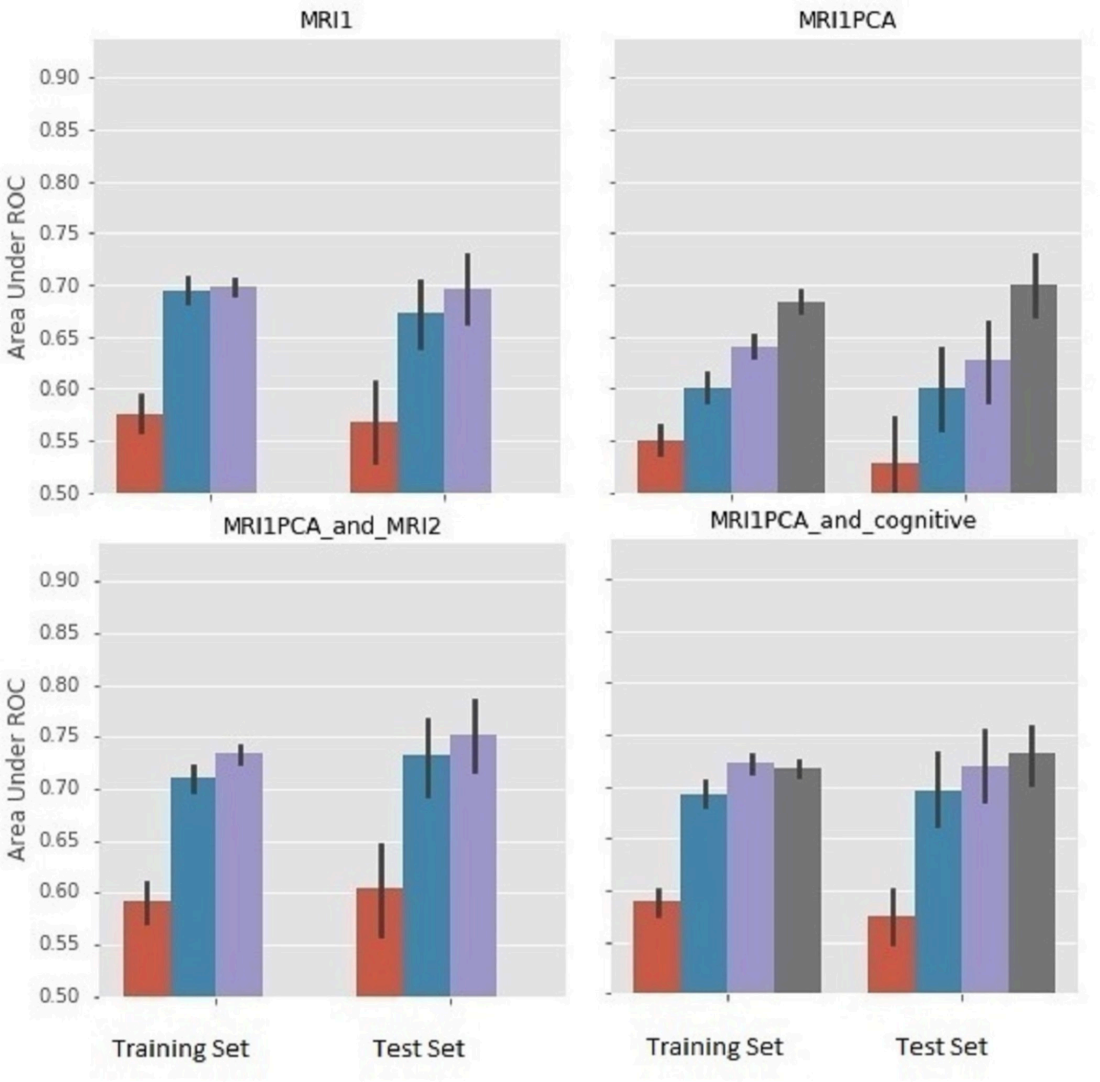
Comparison of different MRI representations & combining baseline MRI data with cognitive or MRI atrophy data. Color coding for bars: red–CART, blue–gradient boost, GB; purple—random forest, RF; dark gray–support vector machine, SVM. For each plot, bars on the left represent performance using leave-two-out cross-validation on the training set, whereas bars on the right represent performance using an independent 45 person test set. The test set was never seen in training and was randomly selected in that it was the complement to the randomly selected training set. We interpreted results from the test set.
